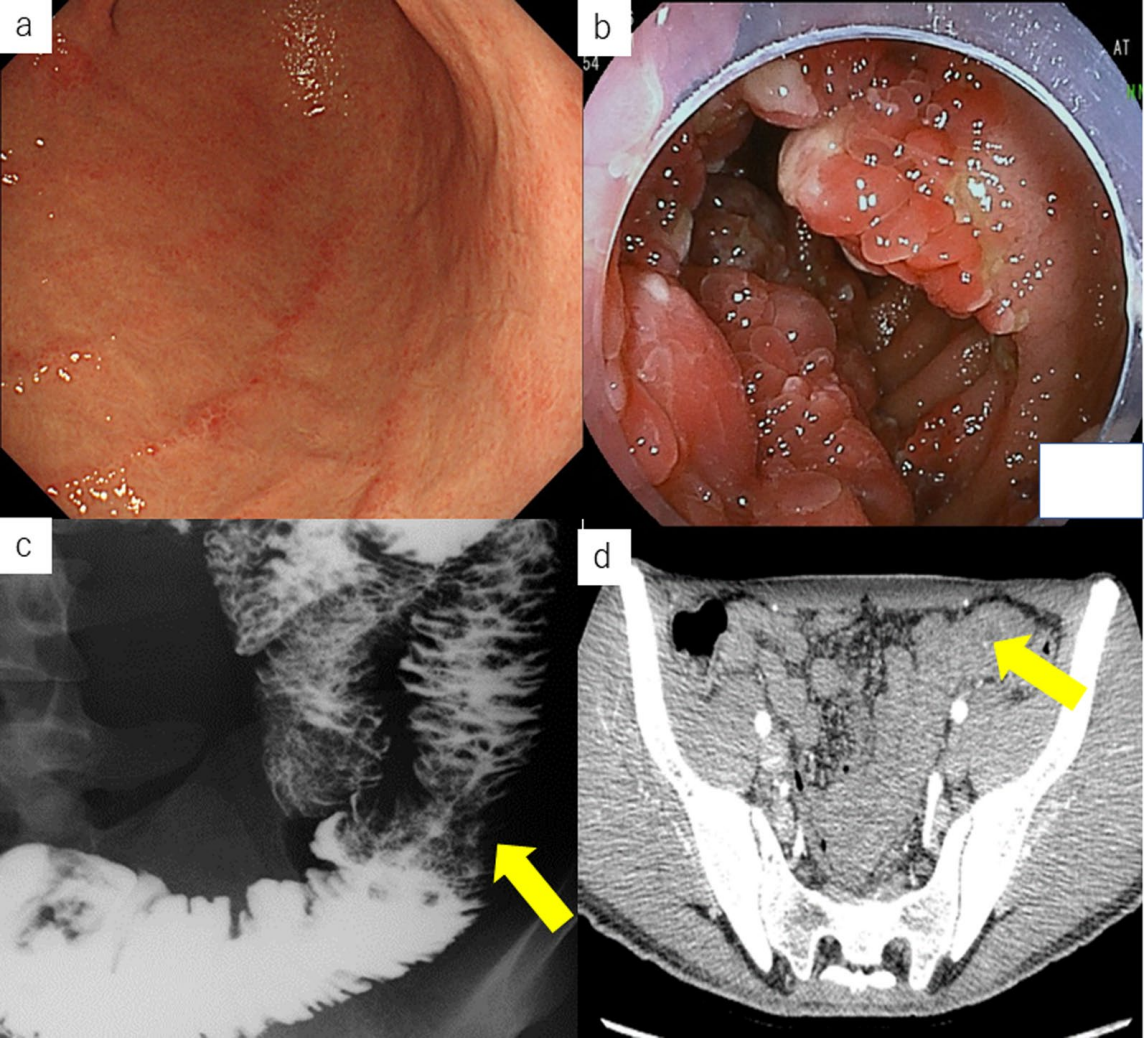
Imaging findings before the first surgery. a Esophagogastroduodenoscopy reveals no lesions in the stomach. b Capsule endoscopy reveals multiple polyps in the jejunum. c Small bowel series reveal multiple translucencies in the jejunum (arrow). d Abdominal contrast-enhanced computed tomography reveals diffuse wall thickening of the jejunum (arrow)
